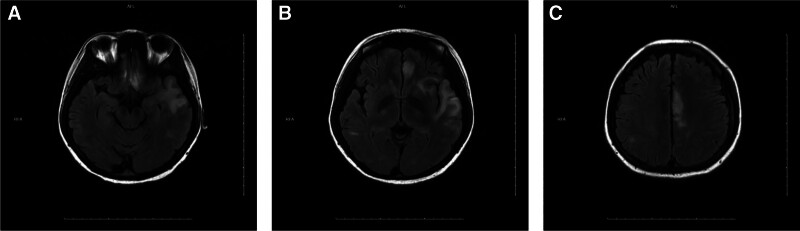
(A–C) November 7, 2023 – MRI reveals patchy areas of T2/FLAIR hyperintensity in the bilateral temporal lobes, basal ganglia, frontal lobes, and parietal lobes. MRI = magnetic resonance imaging.
